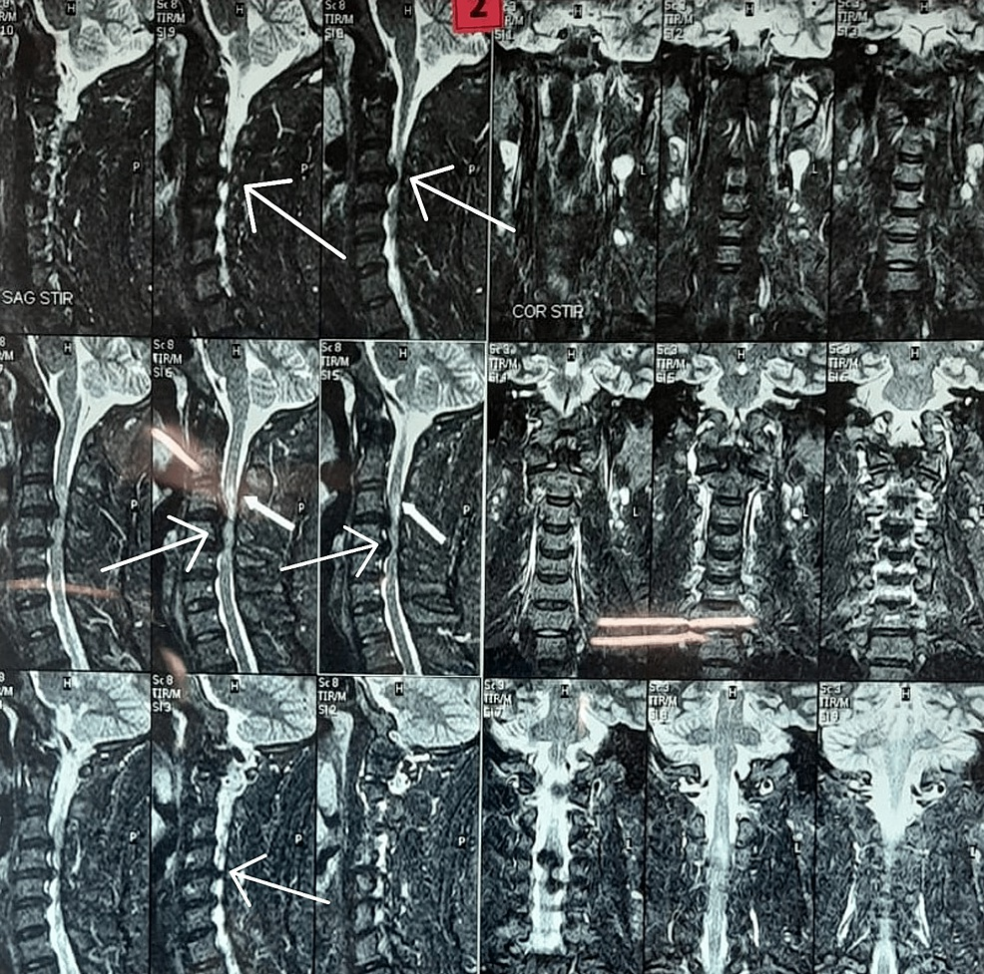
MRI images showing disc bulge Arrows are showing the disc bulges
